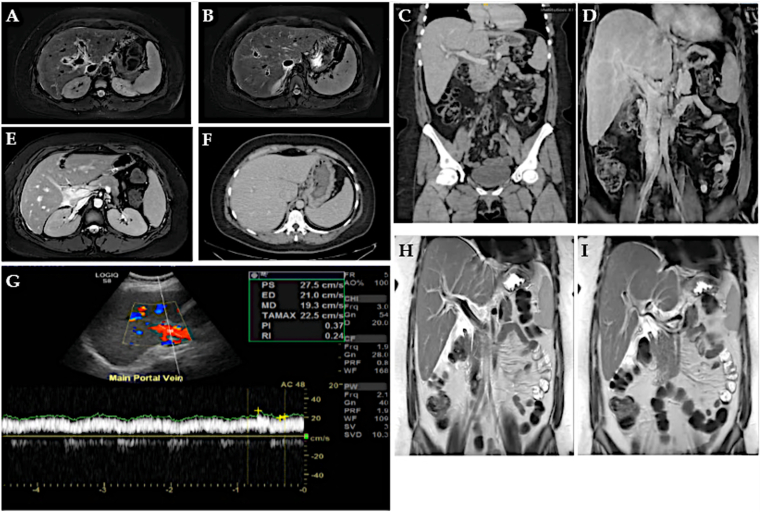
Radiographic findings disclosed mild hepatomegaly on MRI (A, B, D, E) and CT imaging (C and F). Furthermore, magnatic resonance cholangiopancreatography (MRCP) showed a patent biliary tree without evidence of obstruction (H and I), and the doppler ultrasound of portal vein was negative for thrombosis (G). CT, computer tomography; MRI, magnetic resonance imaging.
